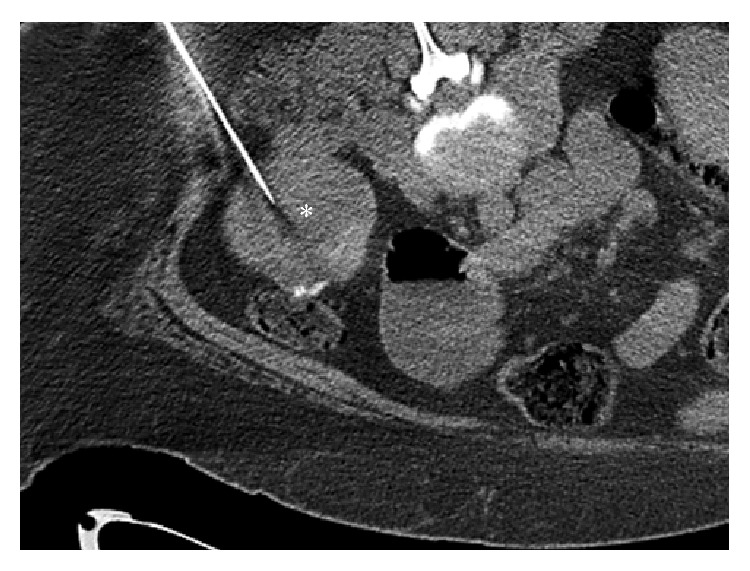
Axial noncontrast CT of the abdomen and pelvis demonstrated a 7.5 × 6.8 cm homogeneous, predominantly exophytic mass arising from the inferior pole of the left kidney. CT-guided biopsy of the left renal mass was performed with a large needle (18 G × 15 cm) (*asterisk*) with the patient in a prone position.
